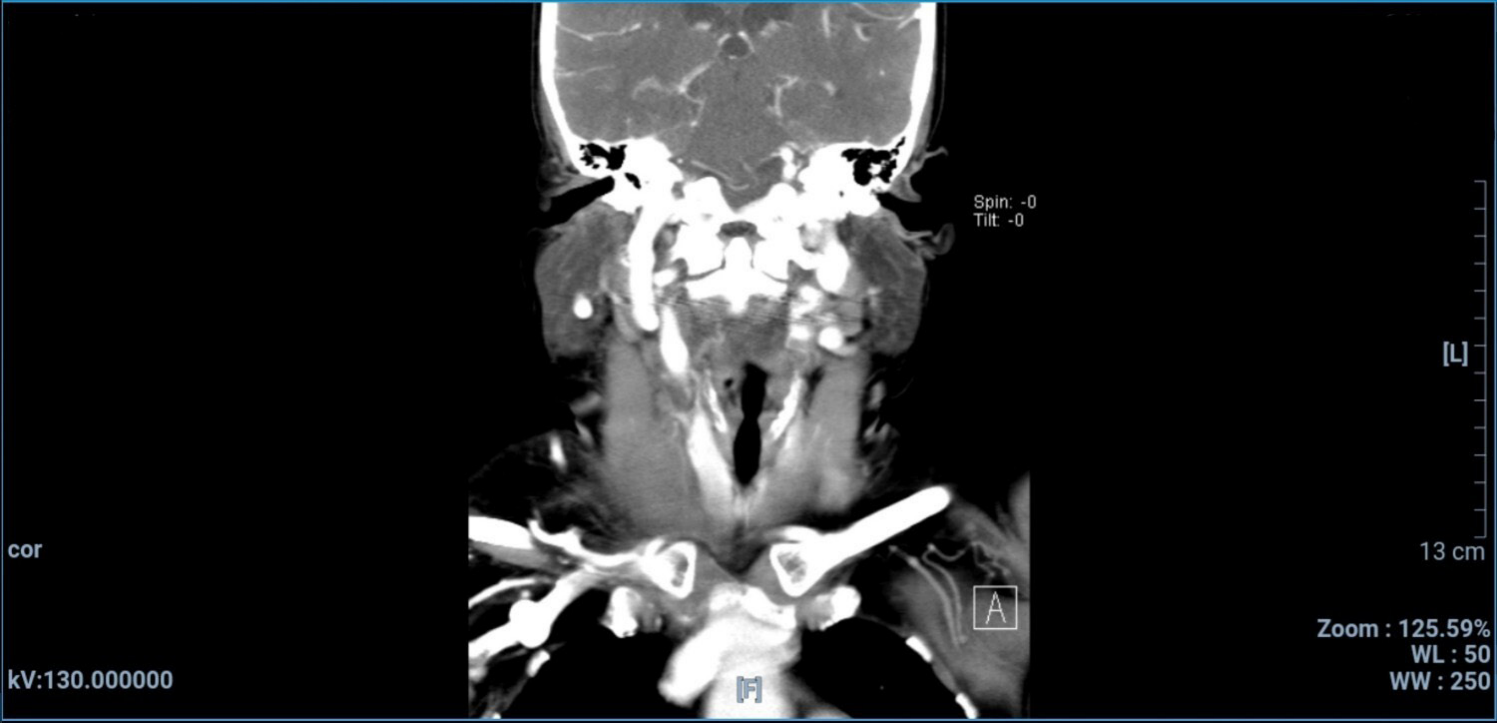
Coronal view of CT scan with contrast showing filling blockage in the right jugular vein.
